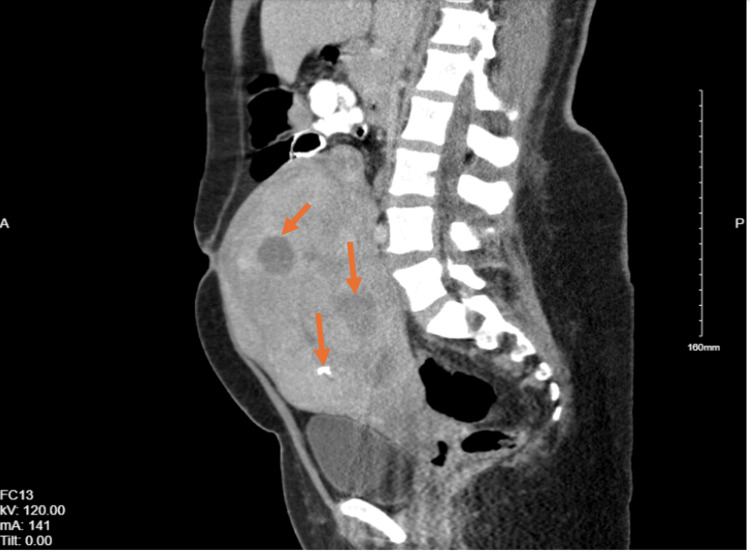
CT-PA demonstrating severely enlarged uterus(10x18x15cm) with multiple fibroids (orange arrows ) CT-PA: computerized tomography of pulmonary arteries
